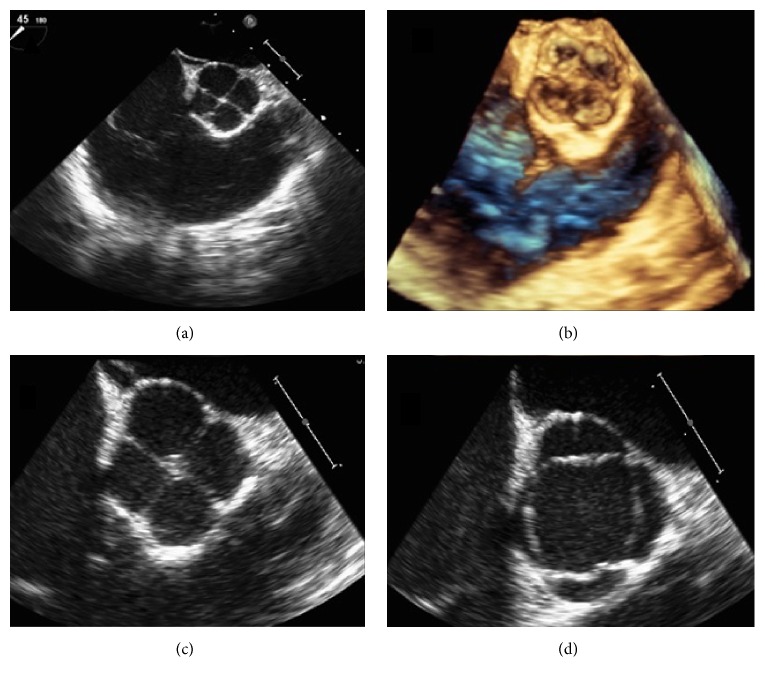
Transesophageal echocardiogram short axis view of the aortic valve revealing QAV. (a) 2-dimensional and (b) 3-dimensional view of the same image. (c) and (d) showing the zoomed view of the short axis QAV in diastole and systole, respectively.
